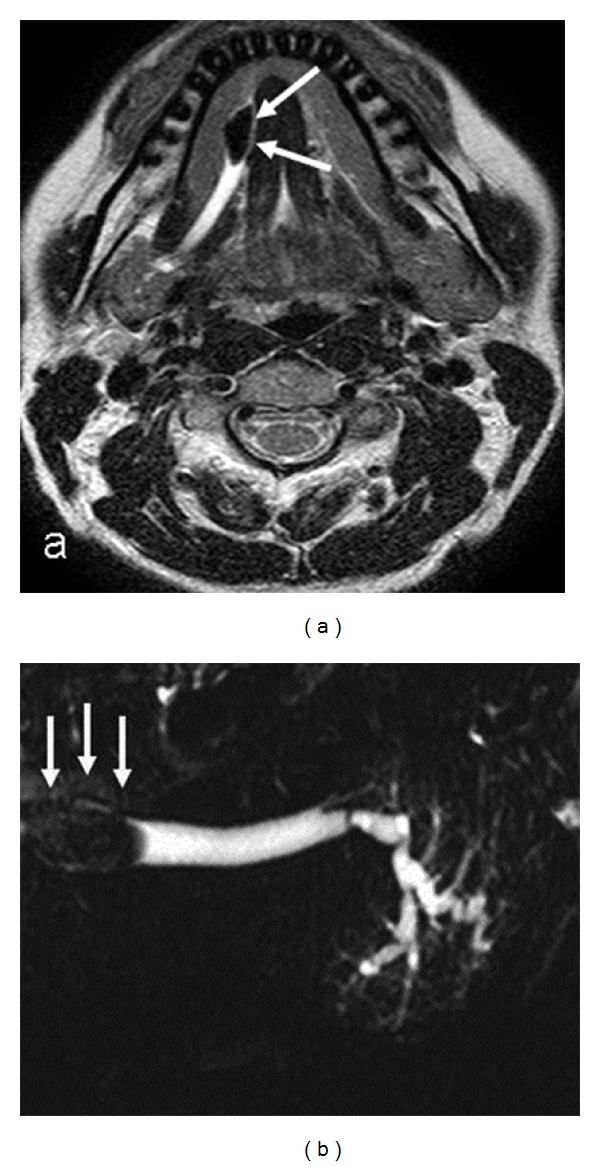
Axial T2-weighted MR image (a) and MR sialography (b) images show the stone located in the right submandibular gland main distal of the duct.
